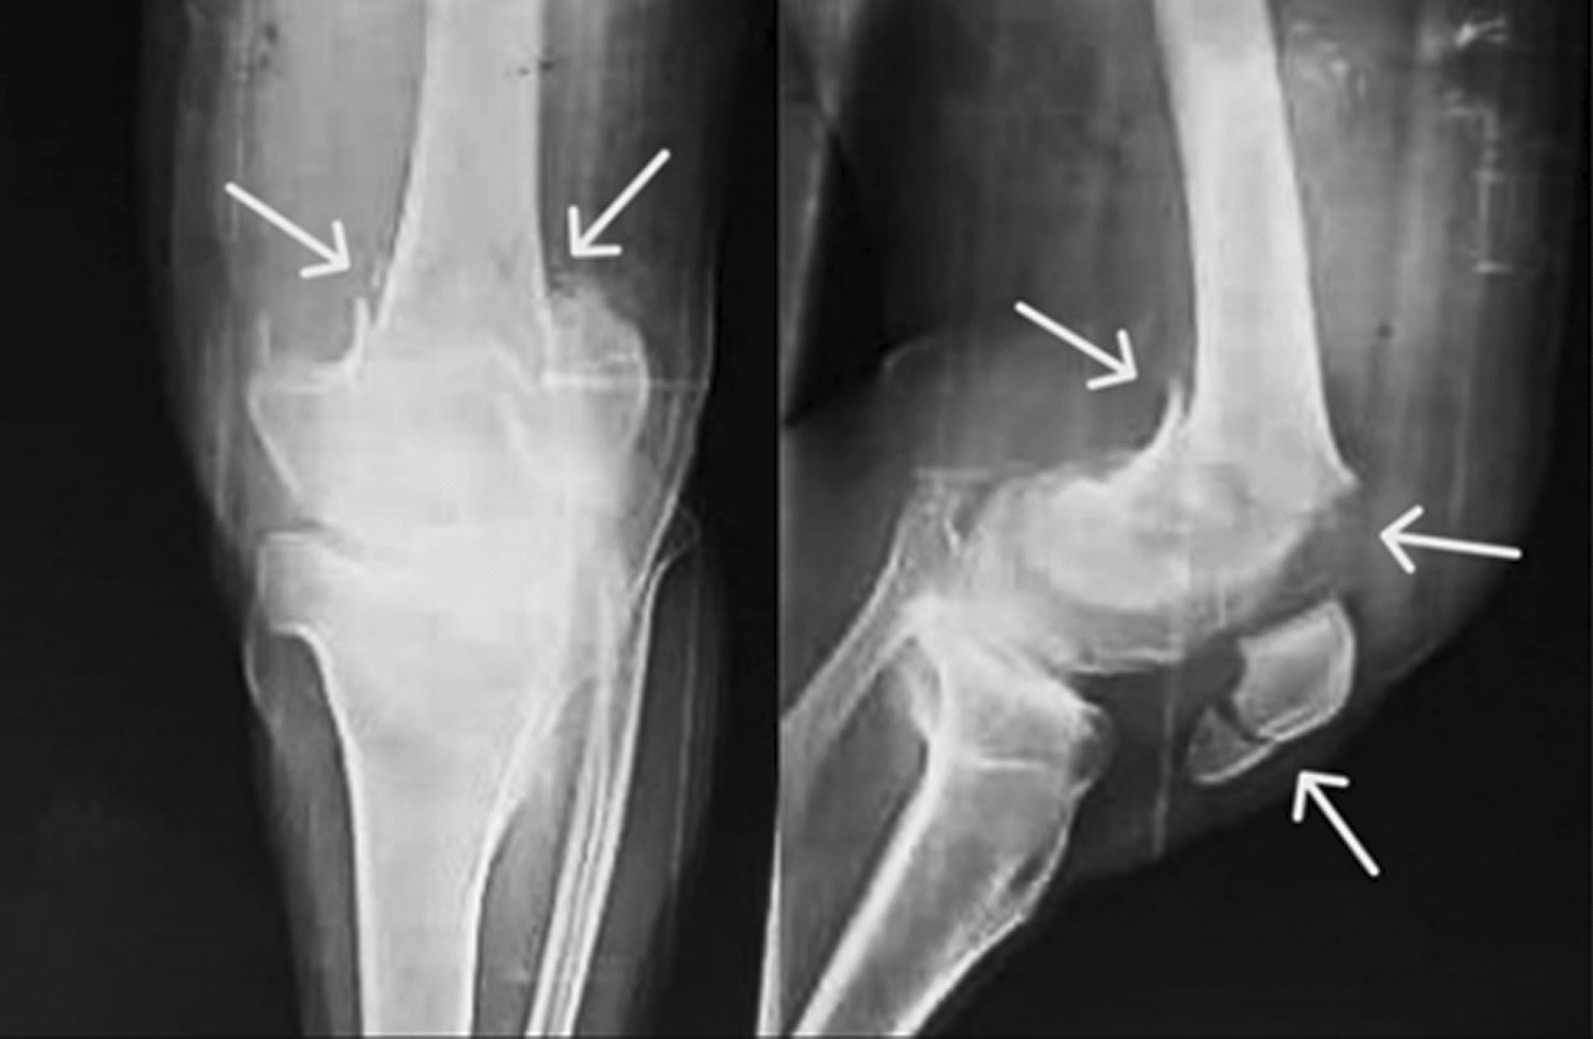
X-ray images showing the distal femoral and patellar fractures as denoted by the arrow indicators
